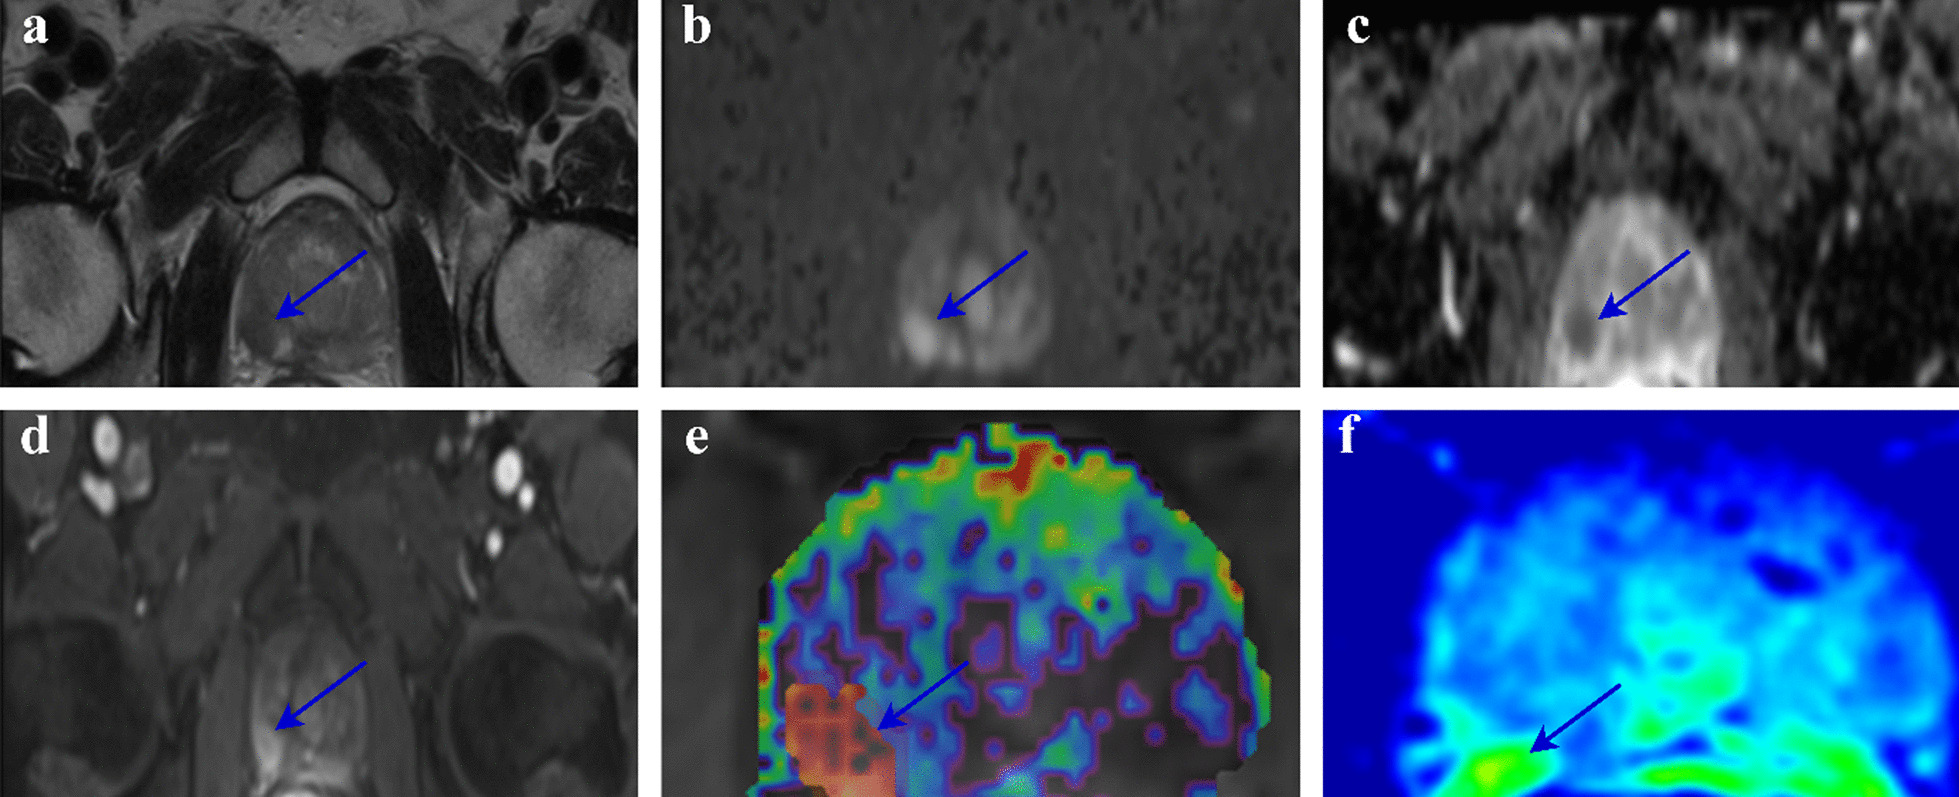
The mpMR images of a 68-year-old man with a DIL in the right lobe (arrows). DIL volume: 0.6 cm^3^, whole prostate volume: 42.3 cm^3^, PSA level: 38 ng/mL, Gleason score: 3 + 4. The DIL is clearly visible as a hypointense lesion on T2W (a), a hyperintense lesion on DWI (b), a hypointense lesion on ADC map (c), an early enhancing lesion on the DCE-MRI (d), Ktrans map (e), and the reconstructed map (f)
